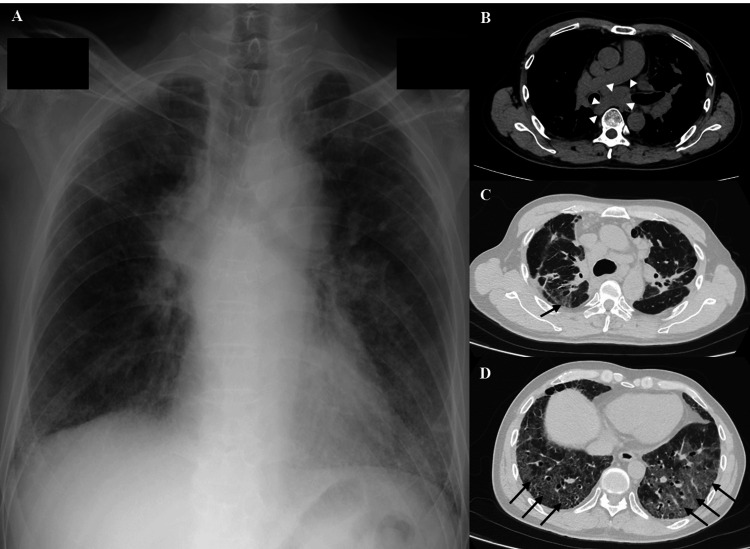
Initial chest radiography and computed tomography (CT) scan (A) Chest radiograph on admission. Diffuse bilateral reticulonodular opacities, predominantly in the lower and mid lung fields, are visible, along with some prominence of the mediastinal silhouette. (B-D) Enlarged mediastinal lymph nodes (white arrow heads), measuring 25 mm in the short axis and 40 mm in the long axis, and diffuse ground-glass opacities (black arrows) are visible in both lung fields. Bilateral lower lobe traction bronchiectasis is also observed, suggesting chronic inflammatory changes.
